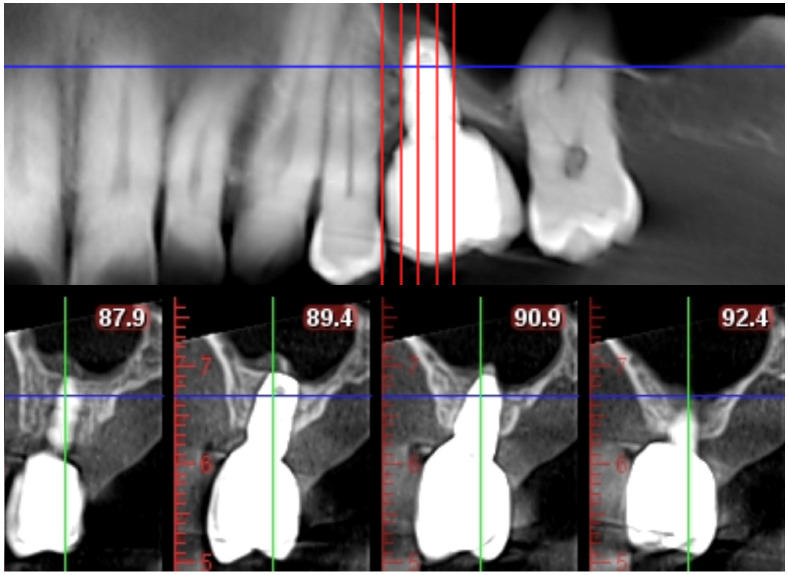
CBCT image of Case #1 at the 45-month follow-up, demonstrating stable implant integration.
